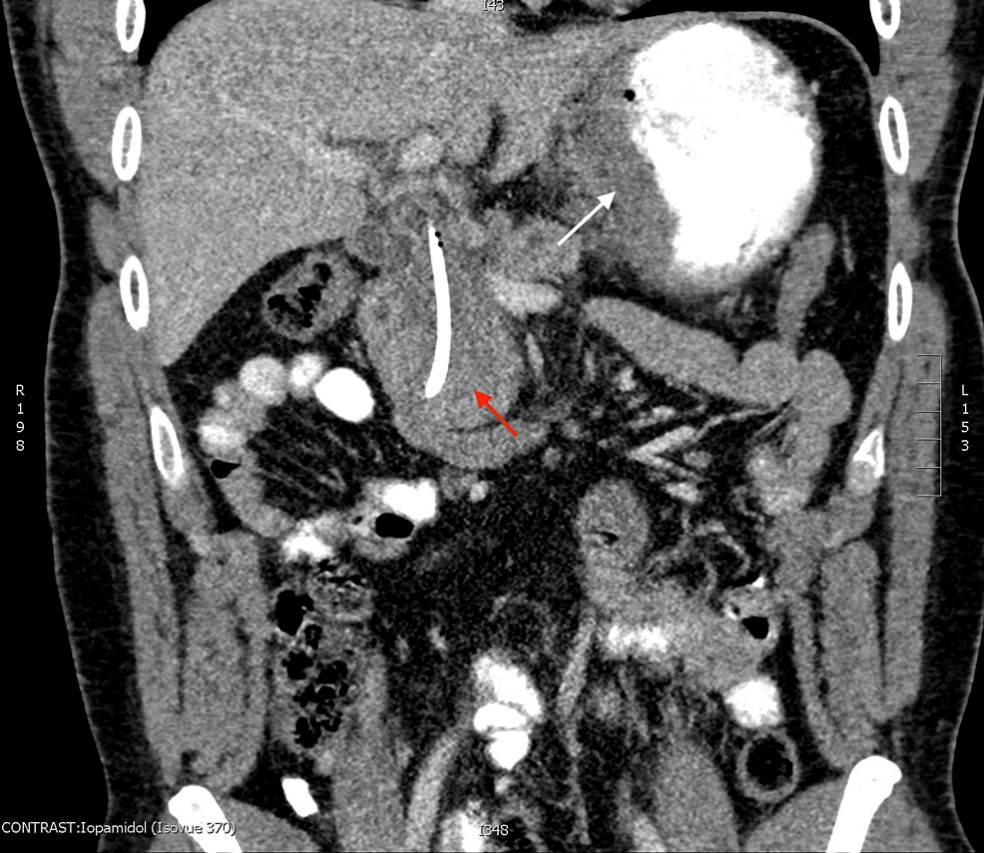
Contrast artifact versus food products versus later discovered gastric mass can be seen in the lesser gastric curvature (white arrow); notably, this was not commented on in the original radiography report. The red arrow shows the pancreatic head mass.
